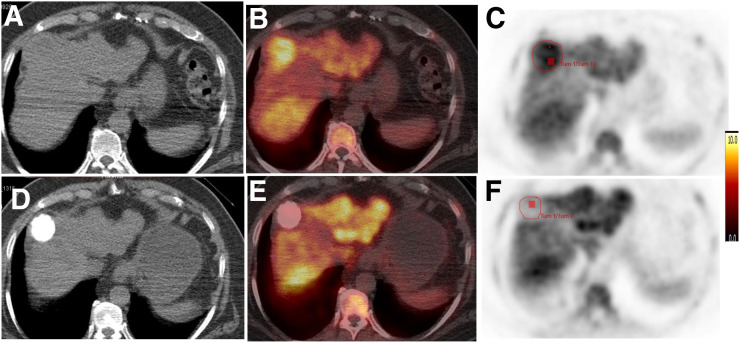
Axial CT, ^18^F-FLT PET/CT, and ^18^F-FLT PET images before (A–C) and after (D–F) TACE show focal HCC lesion (red outline) with increased uptake at baseline and reduction after TACE. Tum = tumor.
